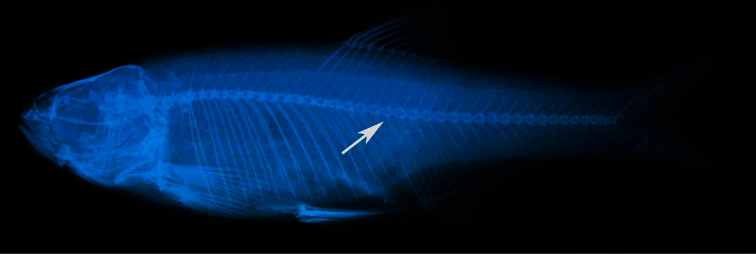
Alburnoides manyasensis* sp. n. Radiograph of a paratype, FFR 01073, 74 mm SL.Arrow shows first caudal vertebra.
